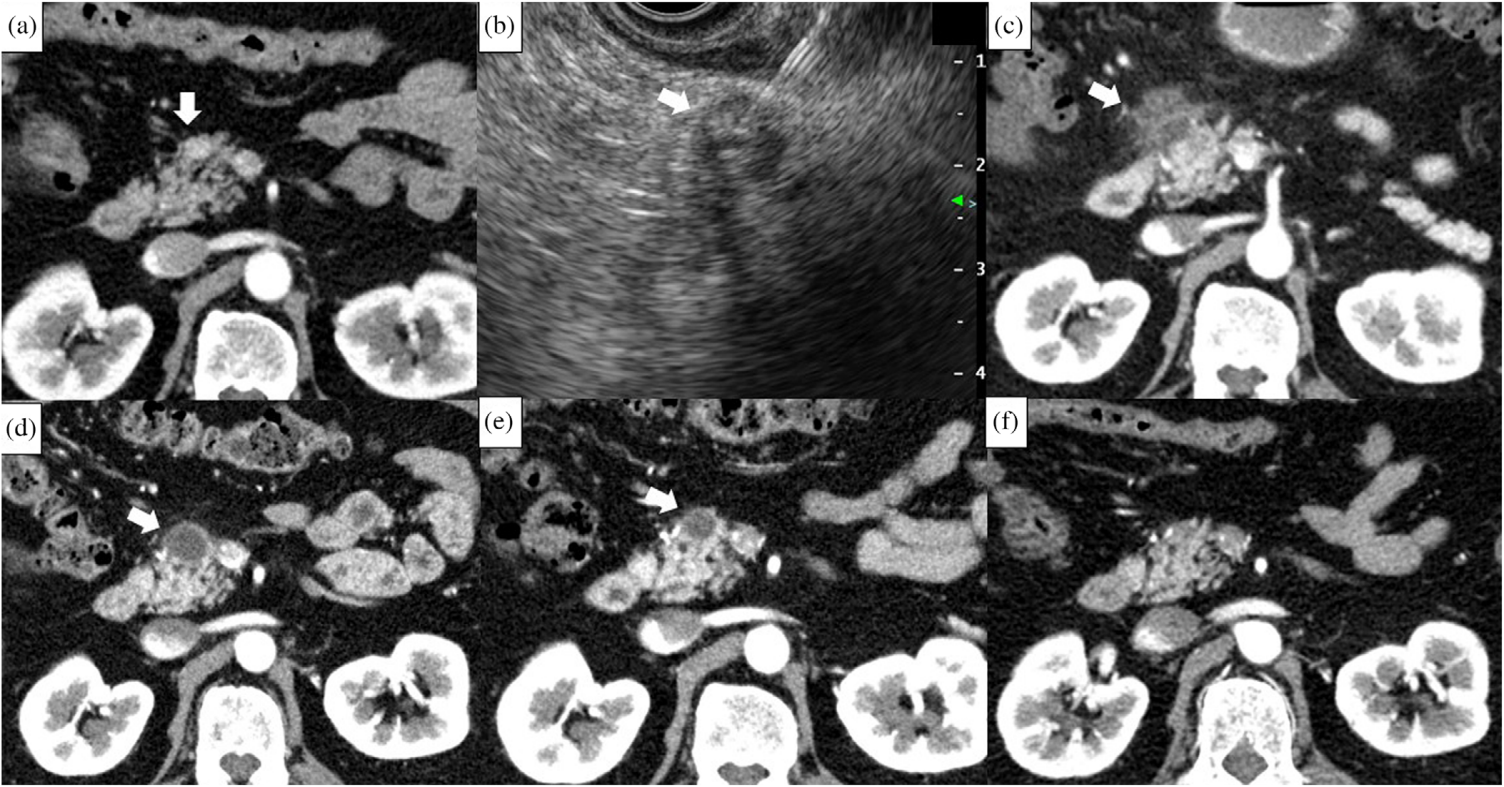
Patient with pseudocyst after EUS‐guided radiofrequency ablation (Patient number 3). (a): An enhanced tumor measuring 12 mm is located at the head of the pancreas on CE‐CT (arrow). (b): A 19‐gauge needle was inserted into the tumor, and EUS‐guided ablation was performed (arrow). (c): CE‐CT performed 3 days after the procedure revealed that the enhancement of the tumor had completely disappeared; however, inflammatory findings had spread beyond the pancreas (arrow). (d): CE‐CT at 1 month reveals the formation of a pseudocyst at the ablation site (arrow). (e): The pseudocyst has decreased in size on CE‐CT at 6 months; however, it is still present (arrow). (f): The pseudocyst has disappeared on CE‐CT at one year. CE‐CT, contrast enhanced‐computed tomography; EUS: endoscopic ultrasonography.
